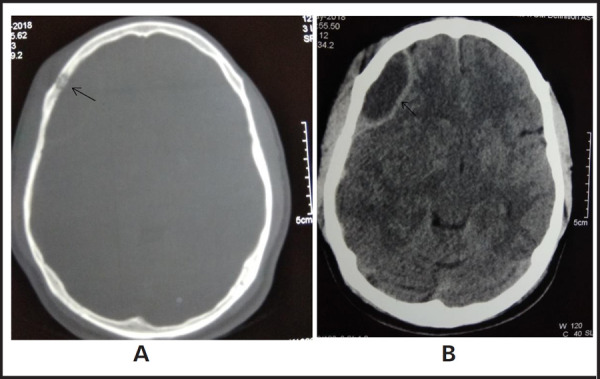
Plain Axial CT scan [(A) - Bone window (B)- Brain window] suggesting bone erosion and epidural and subgaleal collections.
